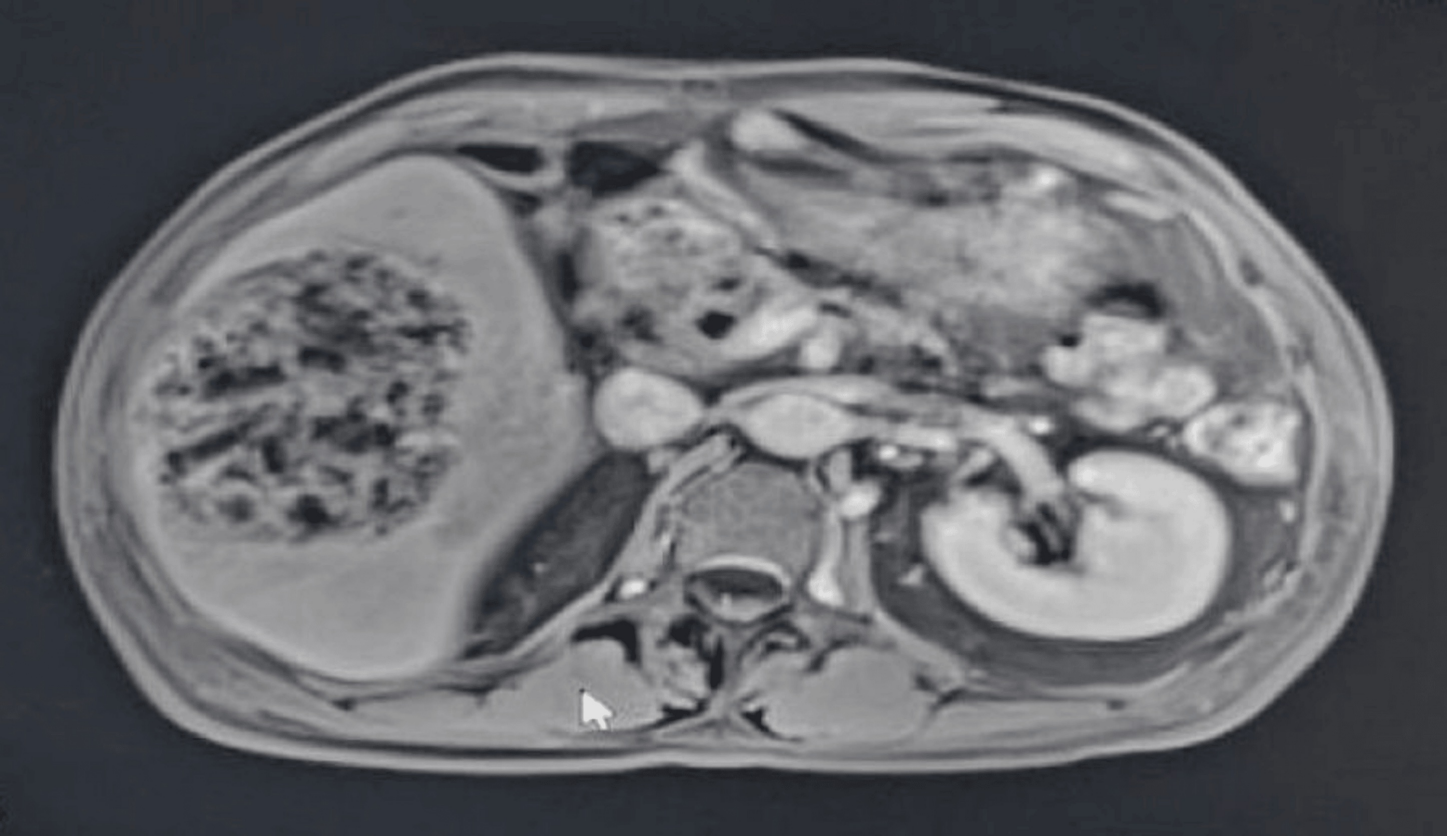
An MRI of the liver showed a large septated cystic hepatic lesion (9.4 x 7.3 x 9.4 cm)
